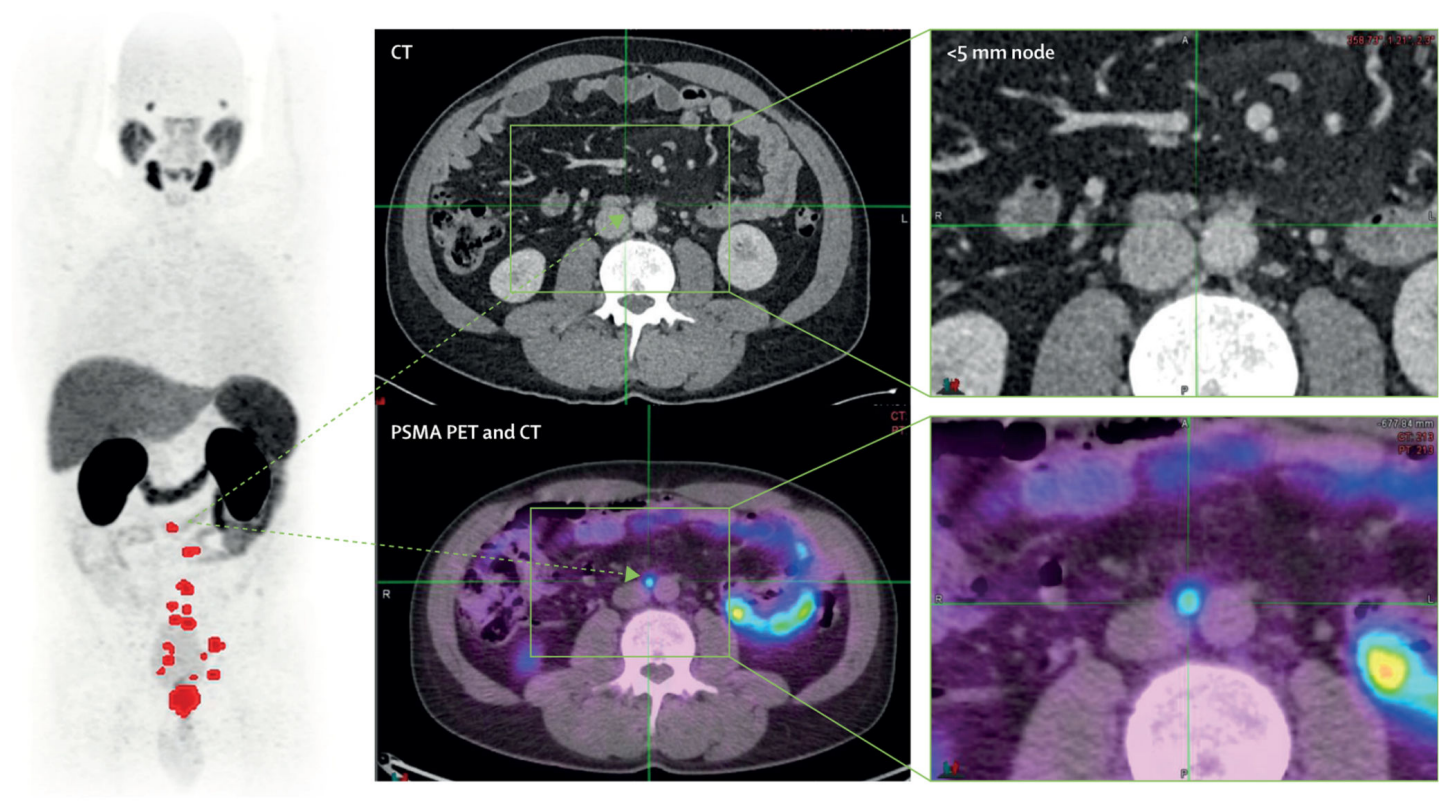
Sensitivity of PSMA PET and CT compared to CT alone in metastasis detection The left-hand panel is a coronal whole-body PET image. Black areas show normal tissue tracer uptake (by the salivary glands, liver, spleen, and kidneys). The red areas represent cancer-related PET tracer uptake in metastatic lymph nodes and the prostate. The upper panels are CT images of lymph nodes with cancer in the para-aortic region. In the lower panels, the addtion of PET to CT clearly shows tracer uptake by low-volume lymph node metastasis, which would be impossible to discriminate in the CT only images. PSMA=prostate-specific membrane antigen.
